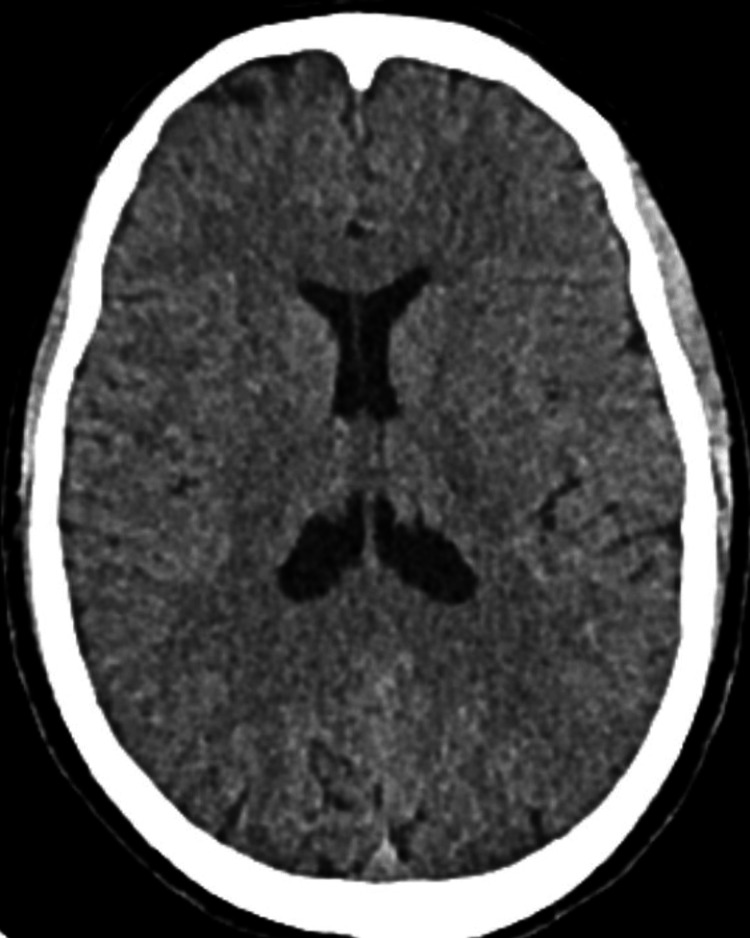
Non-contrast CT of the brain shows no significant abnormalities.
